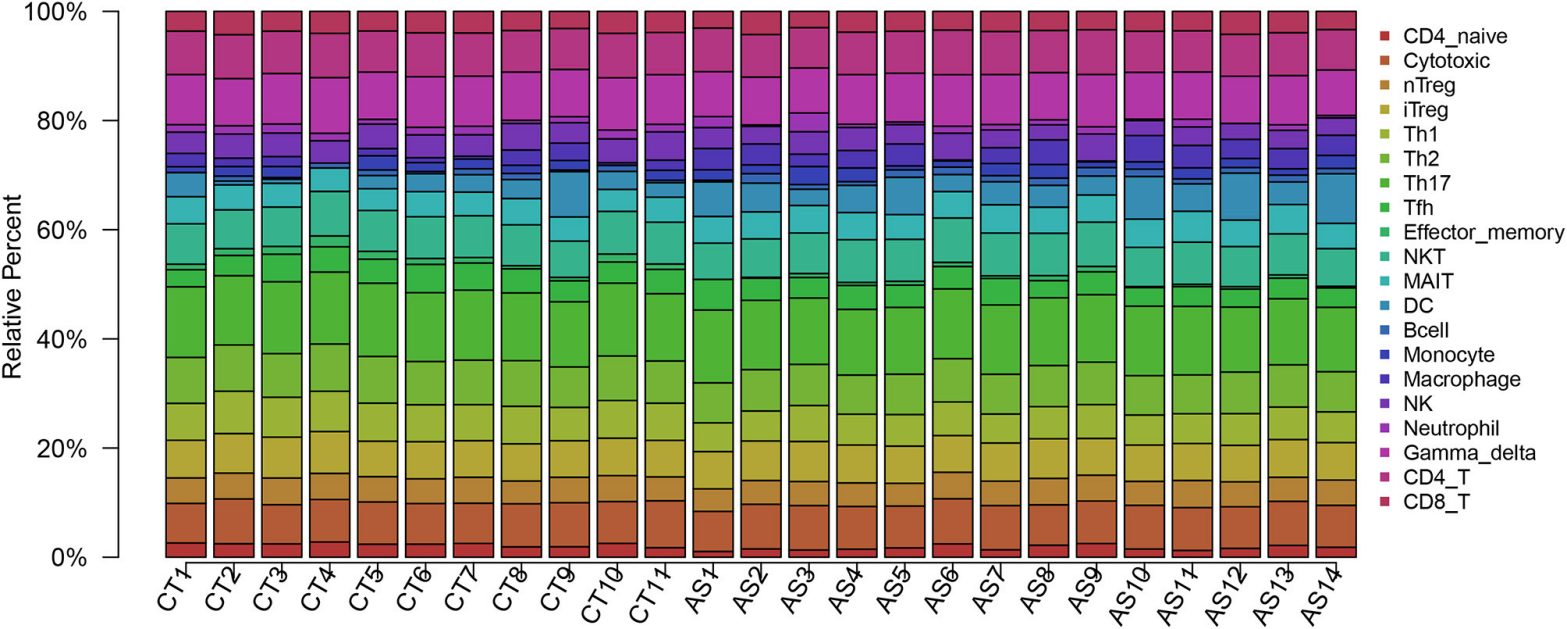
A bar plot of immune infiltration in normal samples and atherosclerosis samples. About 22 subpopulations of immune cells in 25 carotid artery samples [11 normal tissues (CT) and 14 atherosclerotic tissues (AS)] are shown.
